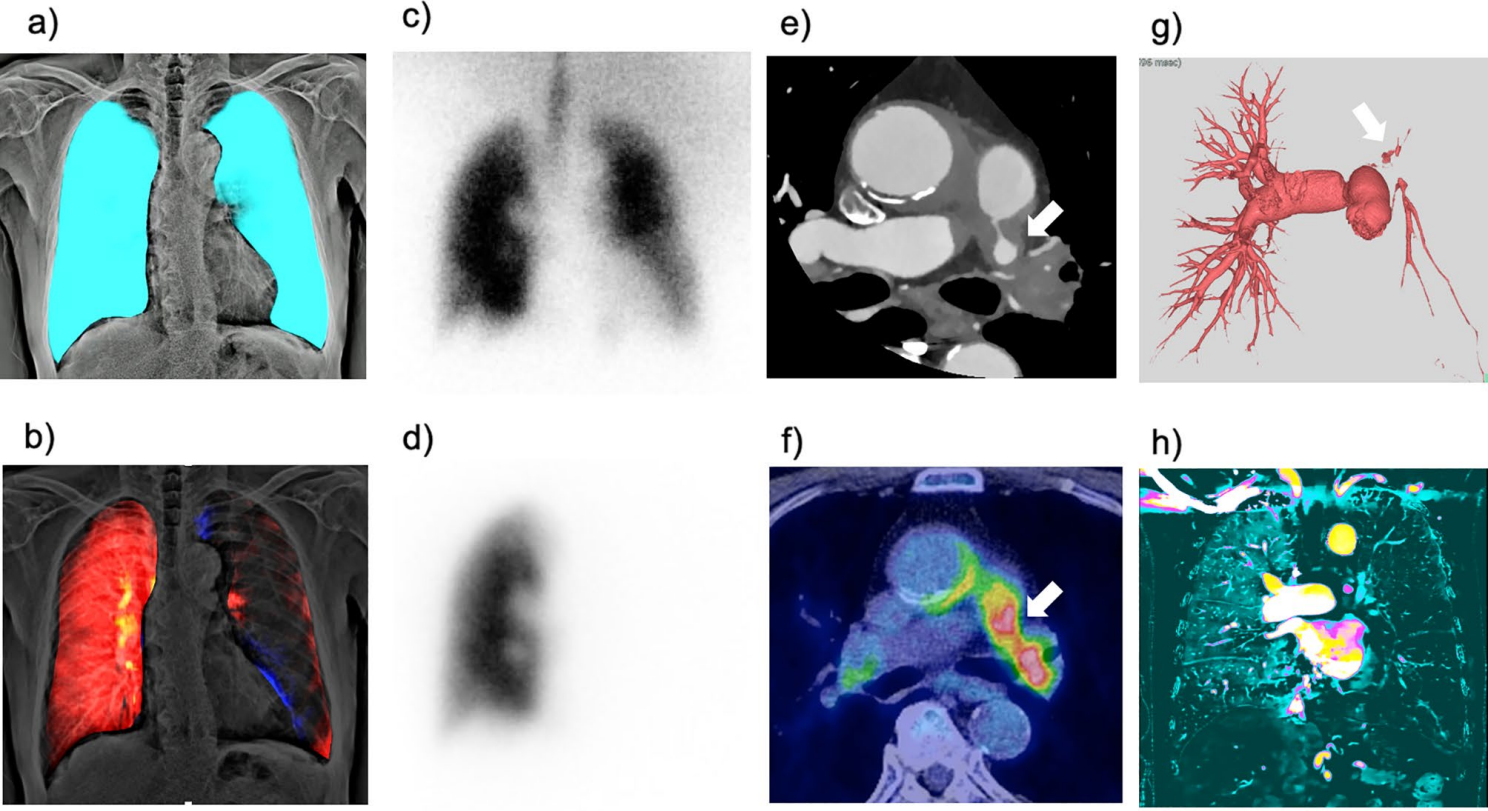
Images of a 74-year-old man with giant cell arteritis. An ipsilateral perfusion defect with normal ventilation (ventilation/perfusion mismatch) in the left lung is observed on ventilation (a) and perfusion (b) images of dynamic chest radiography, similar to the findings of ventilation (c)/perfusion (d) scintigraphy. Computed tomography pulmonary angiography (CTPA) and 18F-fluorodeoxyglucose positron emission tomography (FDG-PET) revealed severe stenosis of the left pulmonary artery due to pulmonary arterial wall thickening (e, g, arrows) with high FDG uptake (maximum standardized uptake value, 7.6) (f, arrow), indicating large-vessel vasculitis. The iodine map created from CTPA also shows a finding similar to that of the perfusion image from dynamic chest radiography (h). *Reproduced with permission from Ref 31 (Yamasaki Y et al. Pulmonary ventilation–perfusion mismatch demonstrated by dynamic chest radiography in giant cell arteritis. Eur Heart J. 2021;42(2):208–209)
